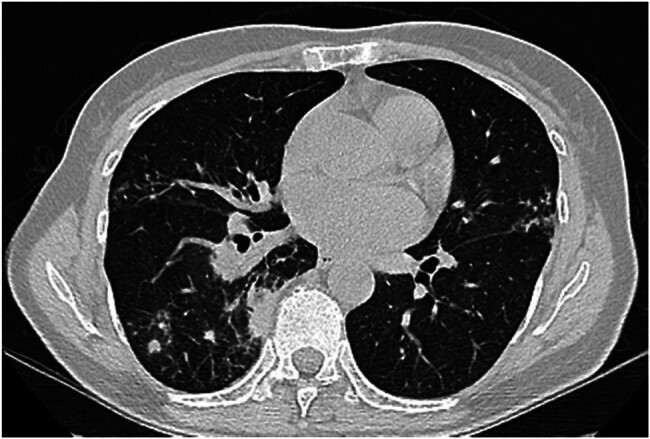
CT image of the patient’s lungs.
